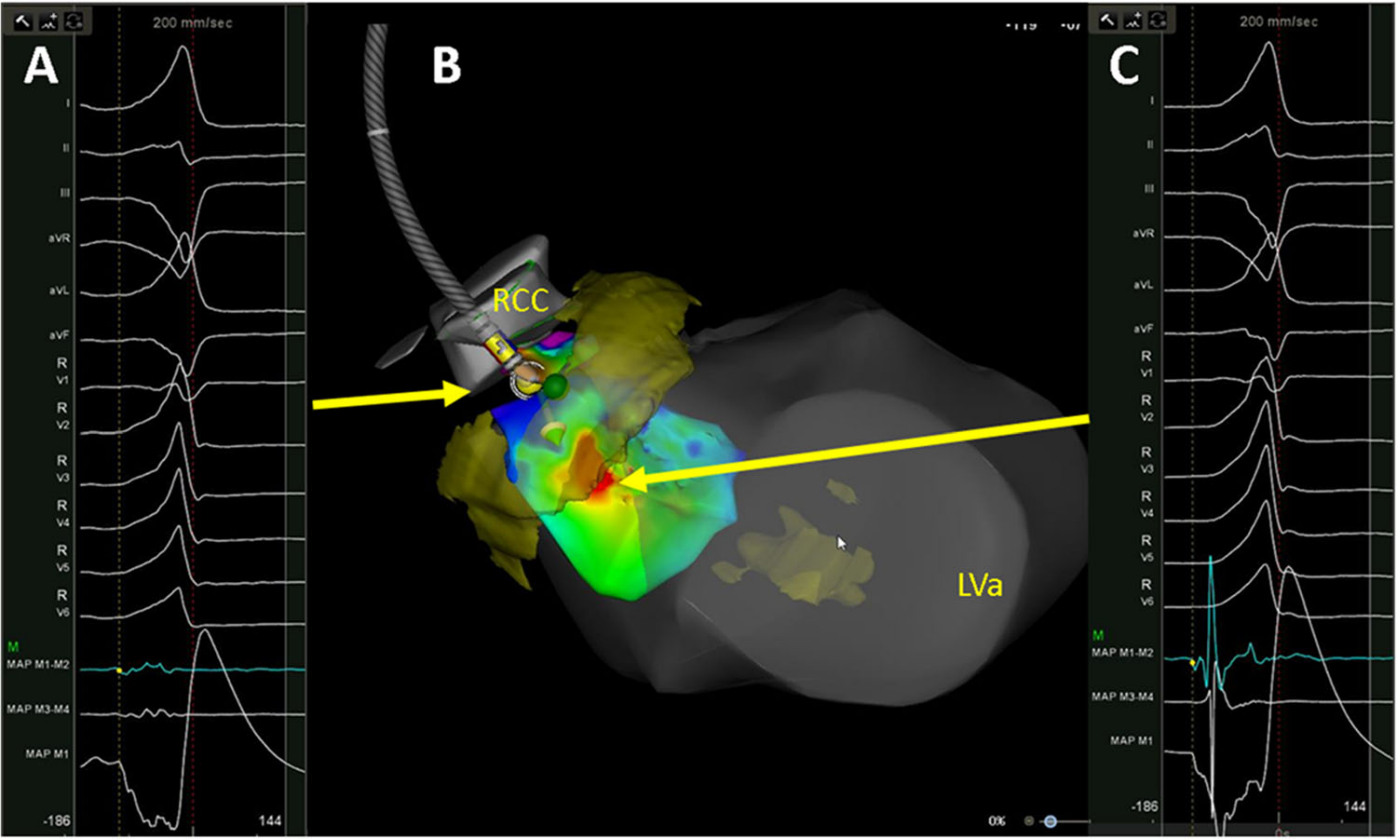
Ablation of parahisian premature ventricular complex. Electroanatomic map with activation data taken from within the left ventricle and right sinus of Valsalva is shown, three‐dimensional reconstruction and overlay of the late‐gadolinium enhanced cardiac magnetic resonance imaging (Figure 1A) is shown in yellow (Pane B). Isochronal maps are shown with early sites of activation shown in red near the parahisian region, located within 10 mm of the his cloud. Presystolic signals from the left ventricle (Panel C) are shown, pace maps in this region were poor and ablation here had no impact on the clinical PVC and resulted in junctional tachycardia. Within the RCC (Panel A), far‐field, low voltage signals at the site of the catheter position shown in Panel B demonstrated similar presystolic timing. There was noncapture in this areas; however, ablation here and at yellow tags resulted in the elimination of the PVC. RCC, right coronary cusp. LVa, left ventricular apex.
